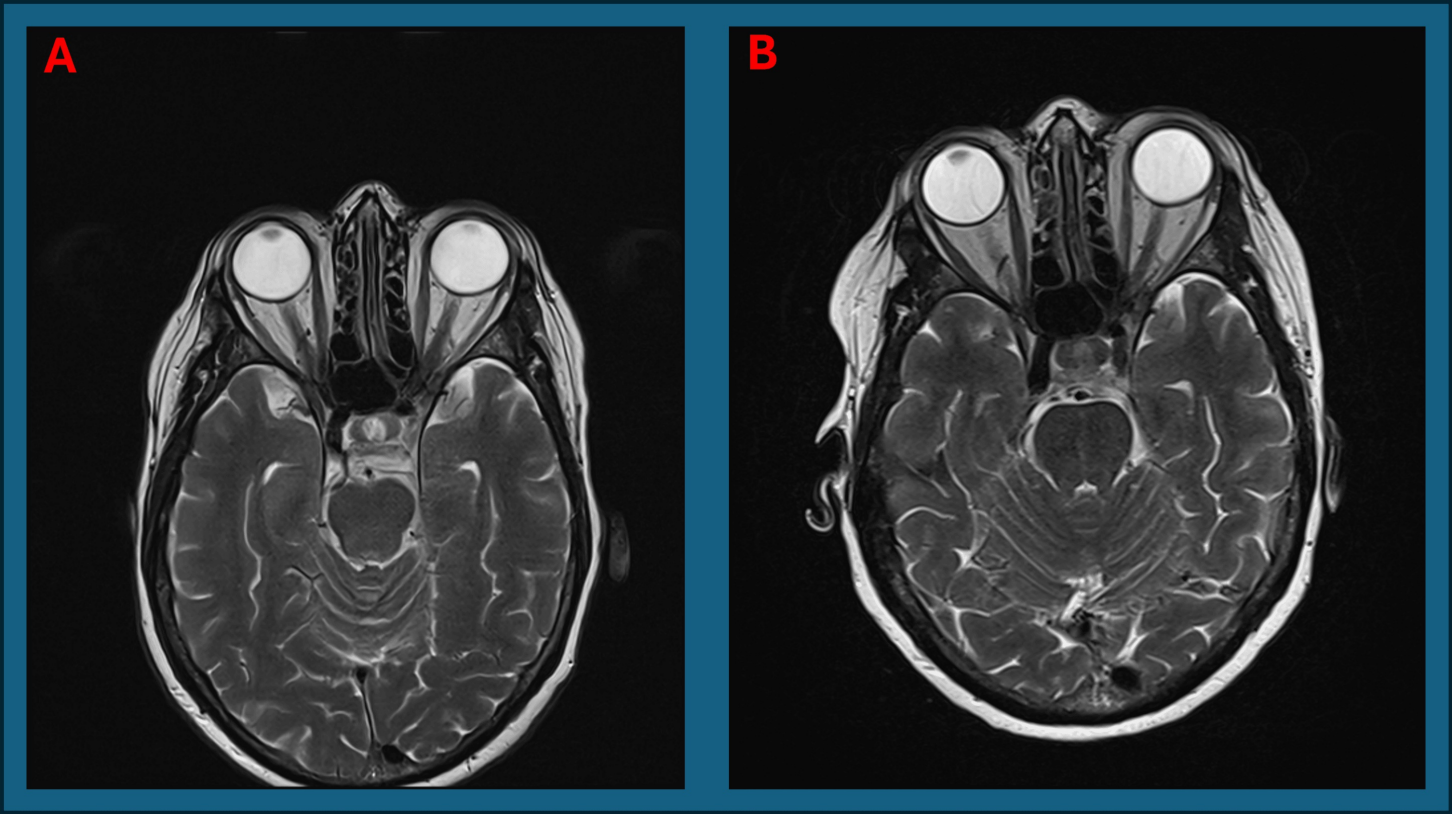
A: Normal axial T2 MRI orbit sequence from second presentation. B: Normal axial T2 MRI orbit sequence on outpatient review after second discharge
